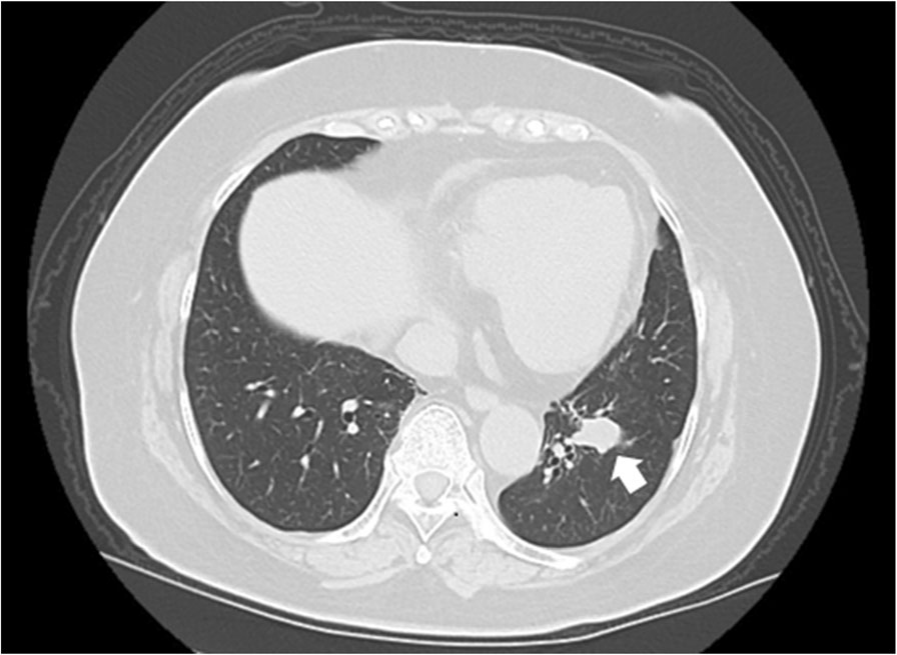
Computed tomography revealed a well-demarcated tumor, 1.8 × 1.3 cm in size, located in the left lower lung (white arrow)
